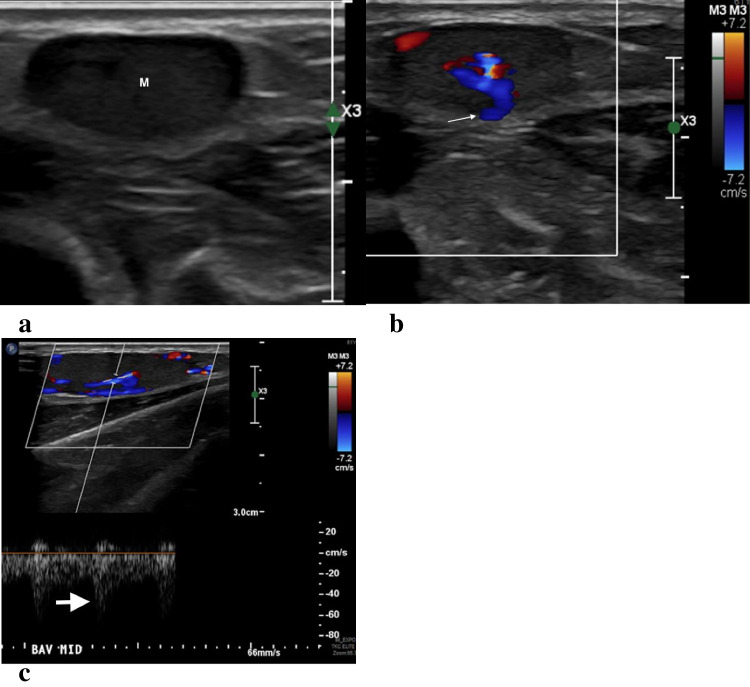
Recurrent intravascular mass in a 61-year-old man with previously treated venous thrombus. Grayscale (a), color Doppler (b) and spectral Doppler (c) images through the basilic vein demonstrate a fusiform hypoechoic mass (M) filling the vein with internal flow (thin arrow). The waveform is arterialized (thick arrow), which is atypical for a venous structure
